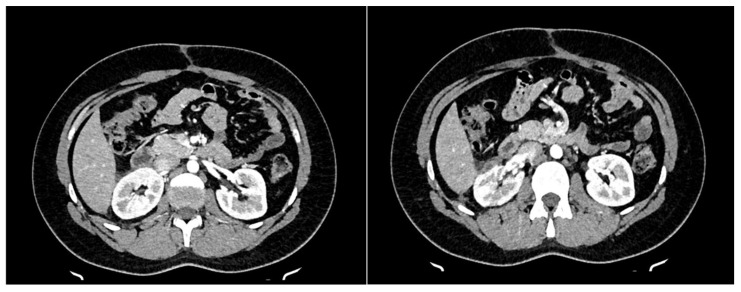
Postoperative CT-Scan showing no signs of local recurrence, in addition, small perirenal seroma can be seen.
